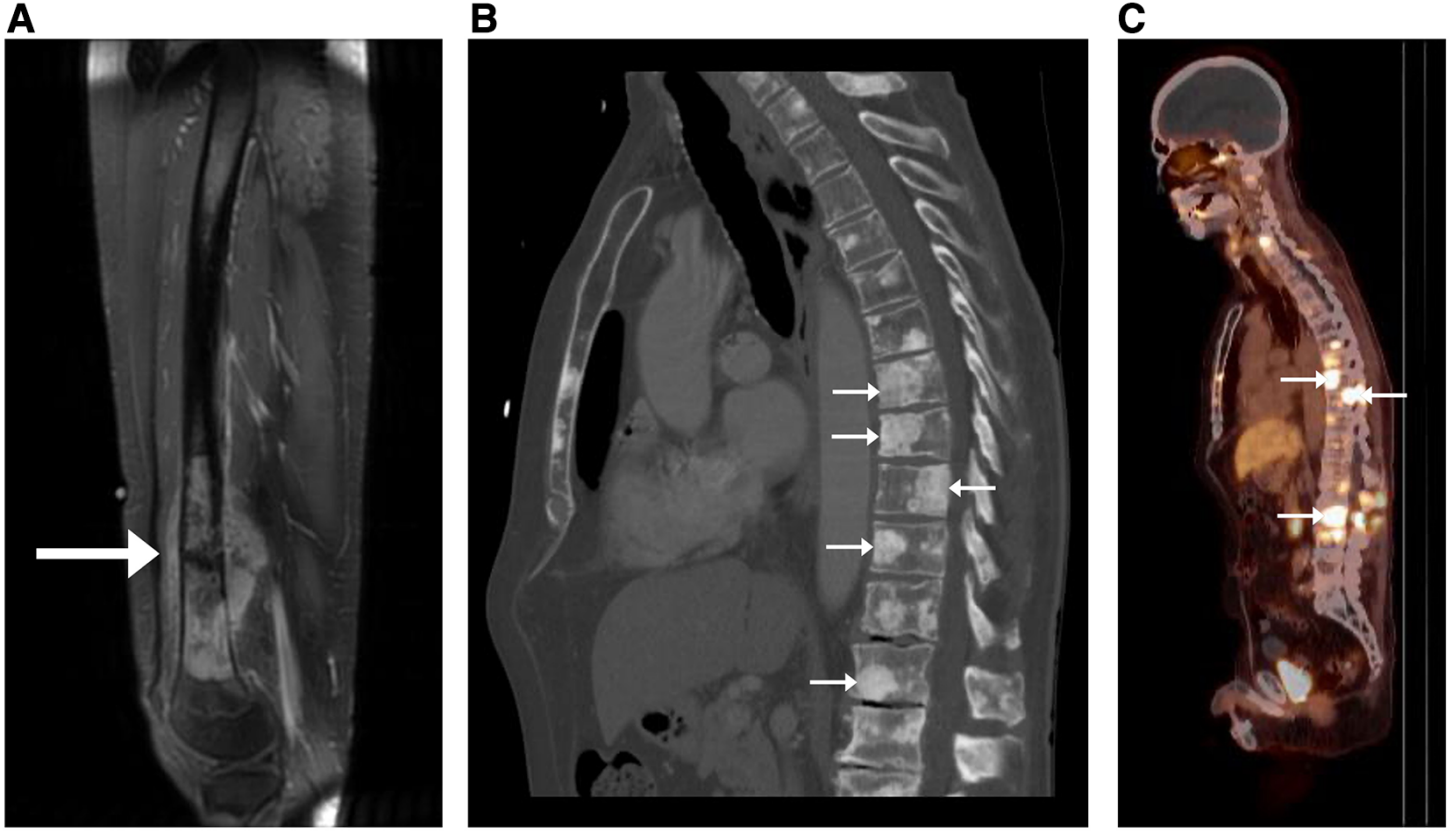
Appearance of malignant bone lesions on different imaging modalities. (A) Sagittal T1-weighted post-contrast MR image with fat suppression of the right femur in a 32-year-old female with biopsy-proven osteosarcoma of the distal femoral metadiaphysis (arrow). (B) Sagittal chest CT with bone windows showing diffuse osseous metastatic disease (arrows) in a 72-year-old male with castration-resistant prostate cancer. (C) Sagittal vertex-to-pelvis prostate-specific membrane antigen (PSMA) PET/CT fusion image showing diffuse osseous metastatic disease (arrows) in the same patient an in (B) 6 months previously. Note that in (Β) and (C), not all metastatic lesions have been annotated with arrows.
